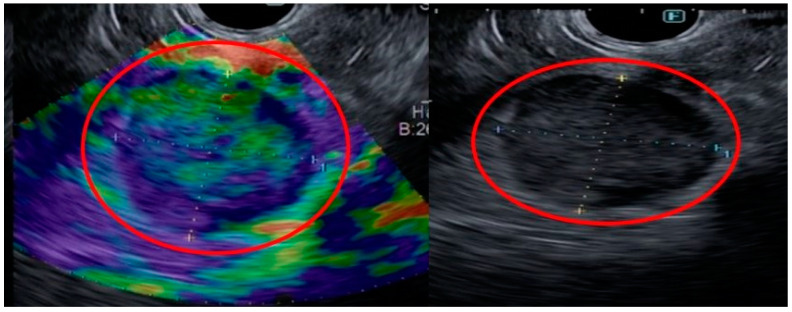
Images from the patient’s endoscopic ultrasound showing a pancreatic mass (red circles) with Doppler (left) and without (right).
